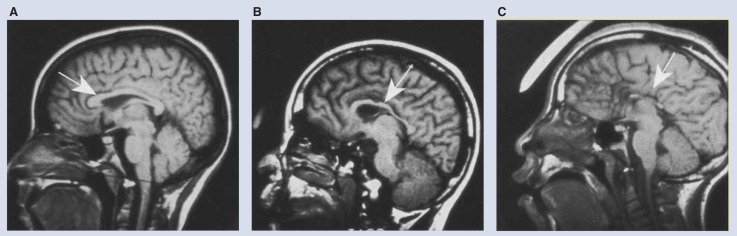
(A) MRI showing the side view of a 14-year-old control subject with a normal corpus callosum, (B) a 12-year-old with FAS and a thin corpus callosum, and (C) a 14-year-old with FAS and agenesis (i.e., absence due to abnormal development) of the corpus callosum. MRI’s courtesy of the authors.
